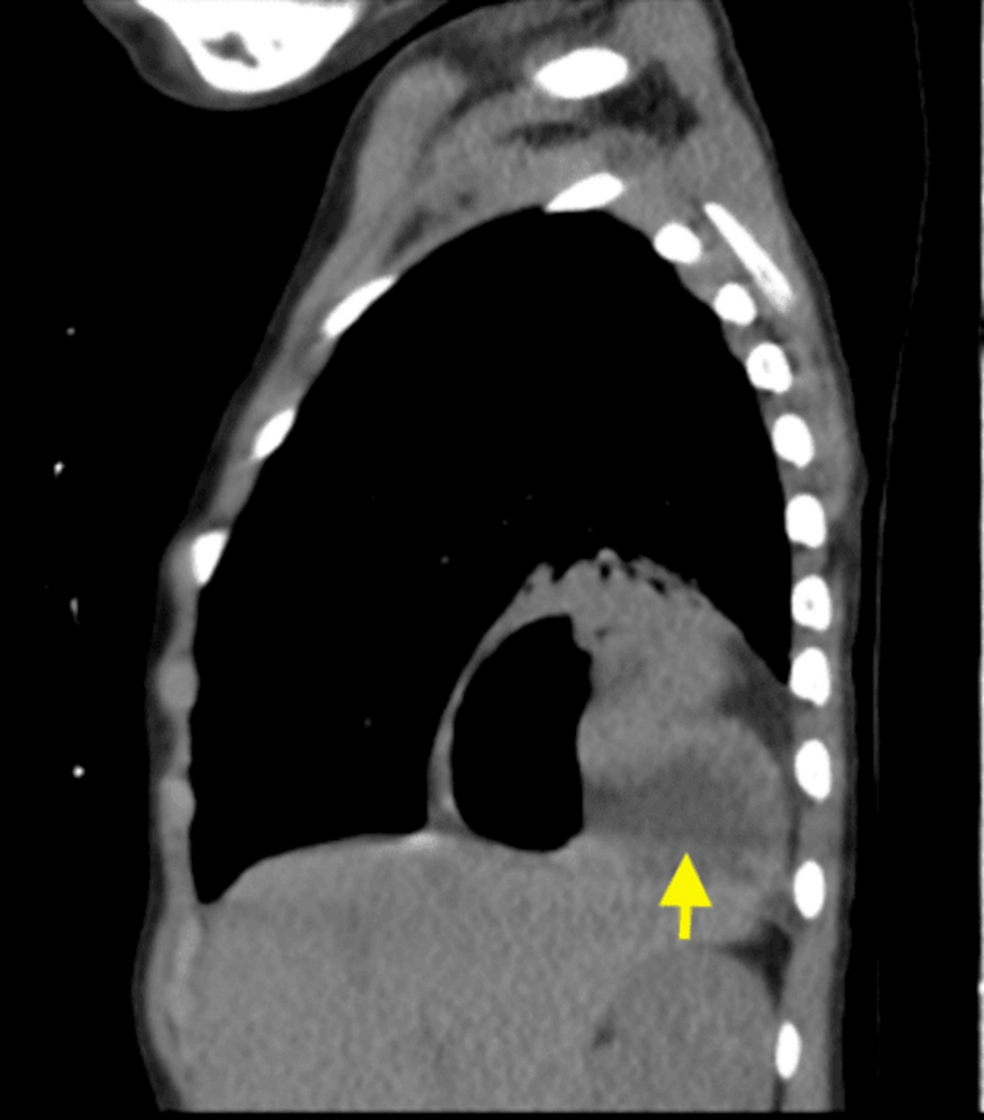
CT chest showed right posterior medial diaphragmatic defect with small bowel and stomach seen herniating into the chest (right diaphragmatic hernia)
